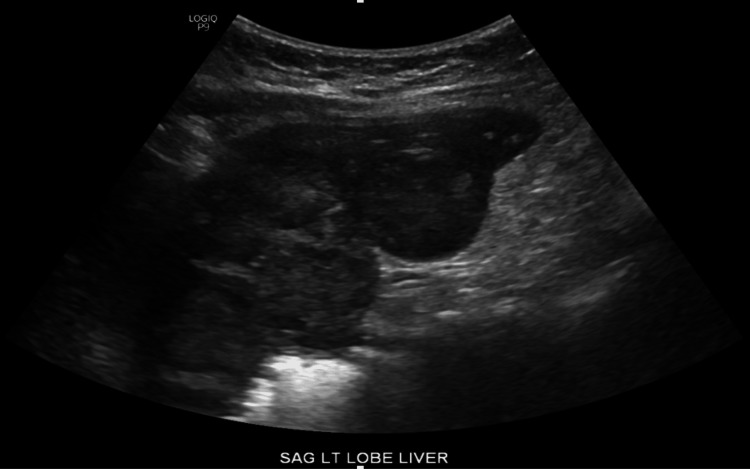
Ultrasound image of liver lesions showing hyperechoic and vascular lesions suggestive of metastatic renal cell carcinoma.
